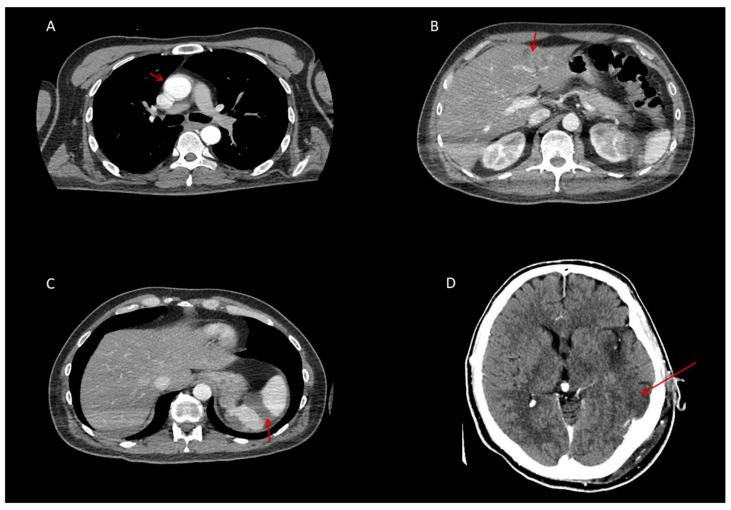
Contrast-enhanced CT and brain imaging (Day 9): (A) abdominal aorta; (B,C) infarct zones in the liver and spleen; (D) left occipito-temporo-parietal ischemic lesion with additional subacute infarctions.
